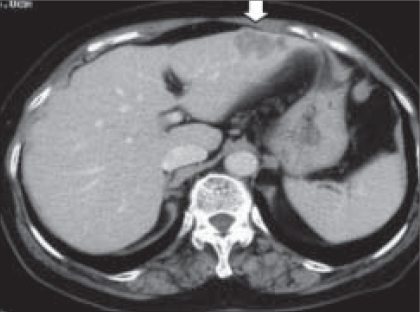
Computed tomographic scan shows a metastatic liver tumour (arrow)
